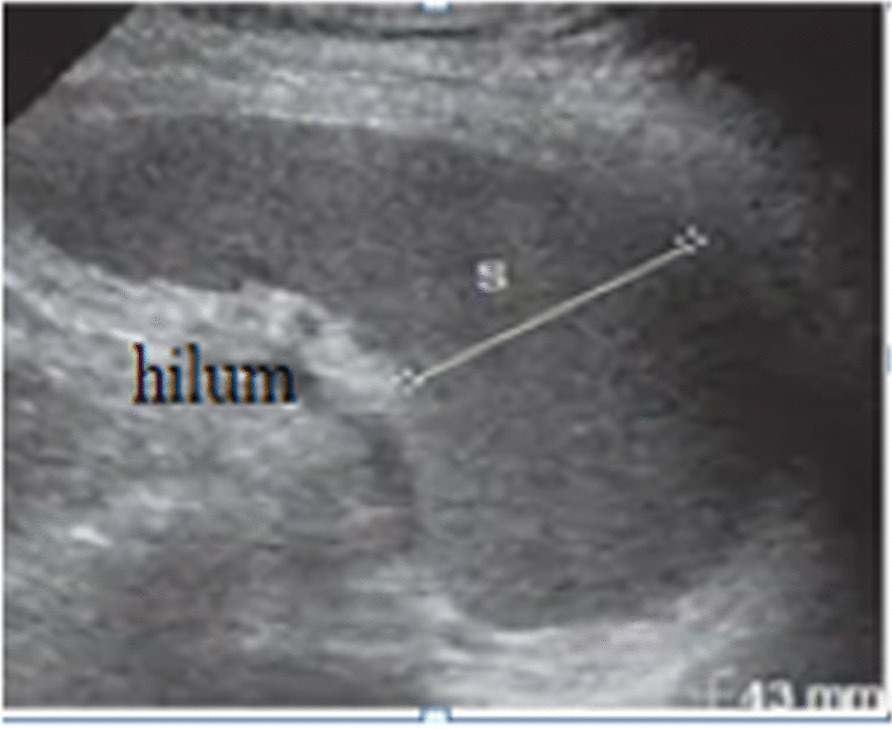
Spleen thickness measured on transverse ultrasound scan (white line)
